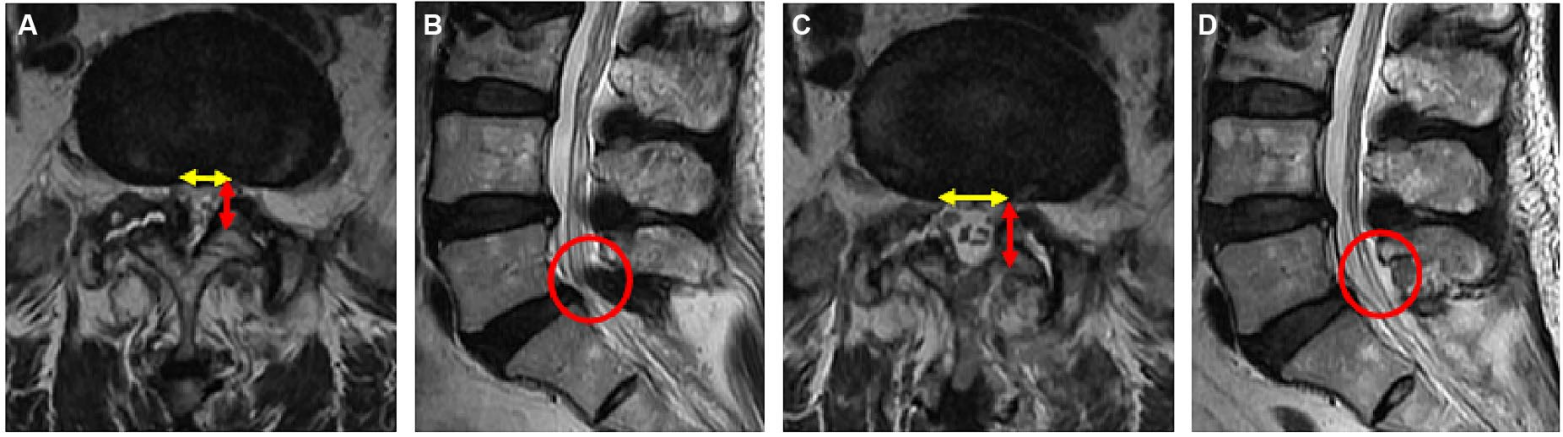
Pre- (A,B) and postoperative (C,D) magnetic resonance imaging (MRI) of the lumbar spine in a 69-year-old female patient. (A) Preoperative axial MR image of L5/S1; (B) preoperative sagittal MR image of L5/S1; (C) postoperative axial MR image of L5/S1; (D) postoperative sagittal MR image of L5/S1.
